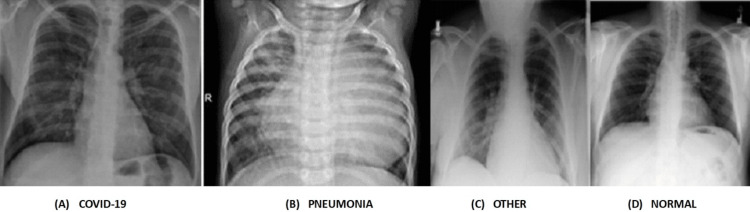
(A-D) Chest x-ray images collected from Kaggle dataset
